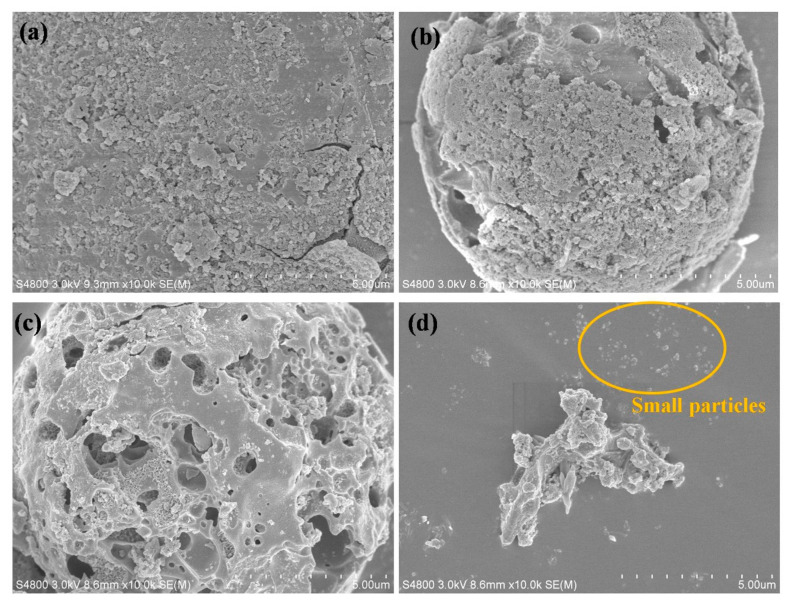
FE-SEM images of OPA particles at different concentrations of NaOH treatment after 30 min ultrasonication: (a) untreated OPA, (b) 1M NaOH treated OPA, (c) 2M NaOH treated OPA and (d) 3M NaOH treated OPA.
